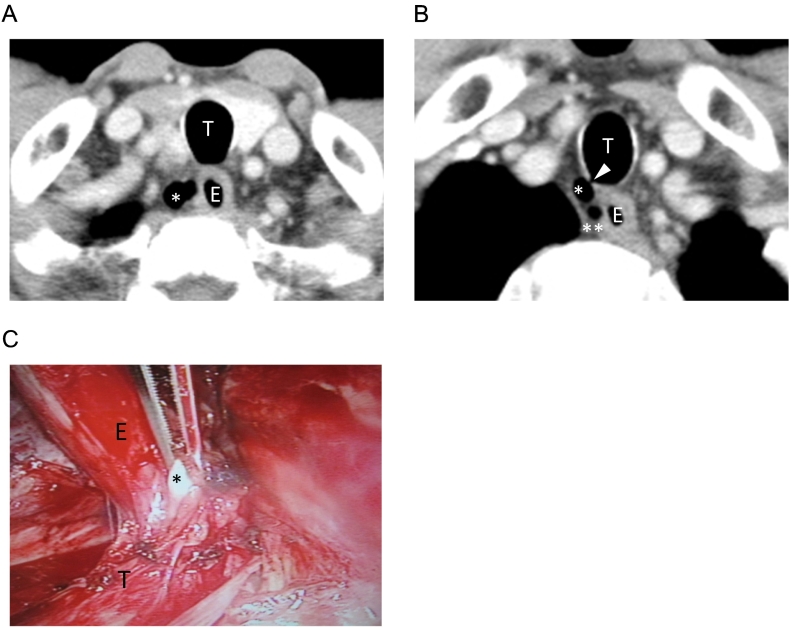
(A) Preoperative CT image of the PTAC in Patient 3 (asterisk). (B) Communication of the PTAC (asterisk) to trachea (white arrow head). An isolated BC was also seen (double asterisks). (C) Intraoperative findings of the BC in Patient 3 (asterisk). E: esophagus, T: trachea.
